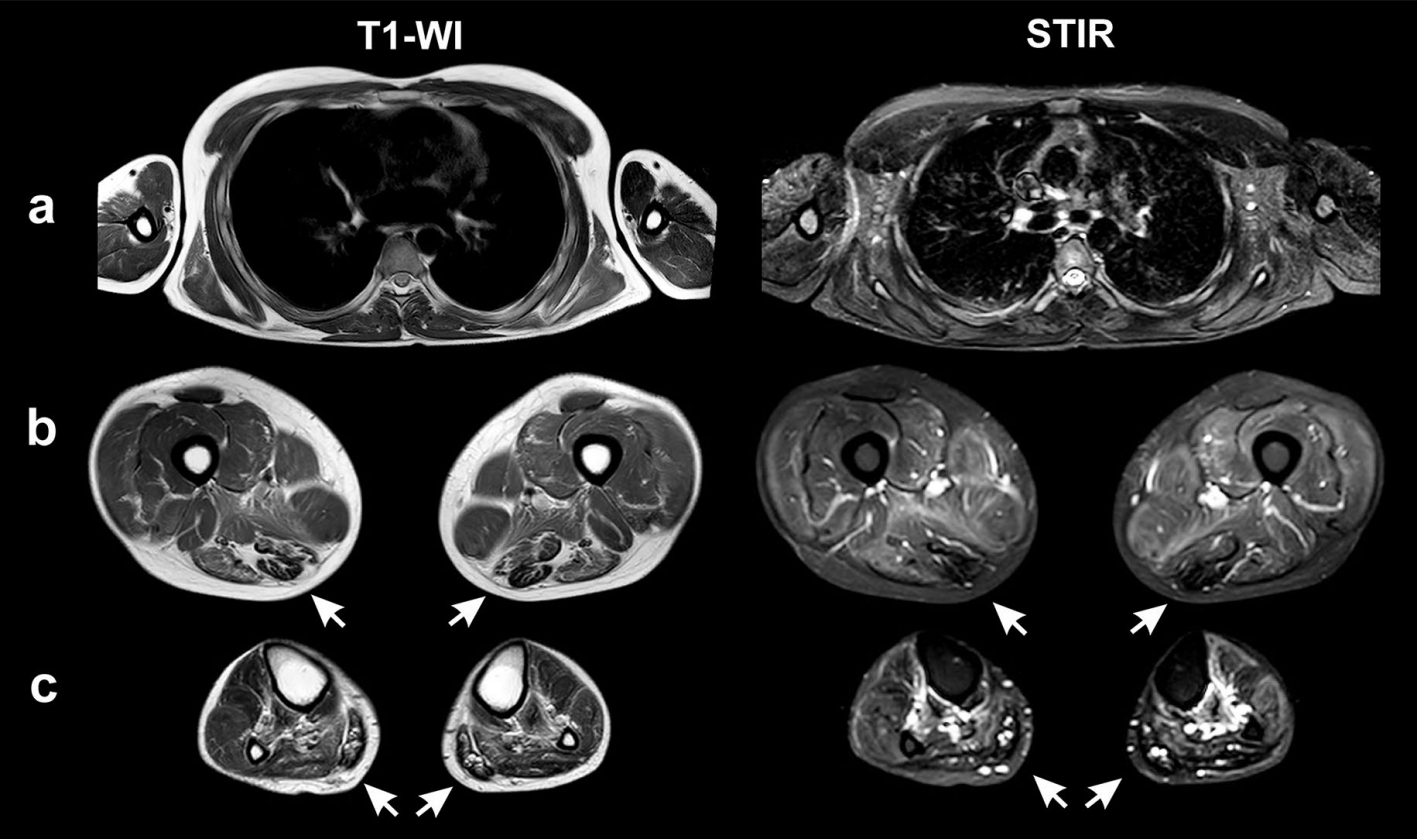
Magnetic resonance imaging (T1-WI, STIR) of the muscles of the trunk and shoulders (a), thighs (b), and lower legs (c). The arrows indicate muscles undergoing fibrosis
